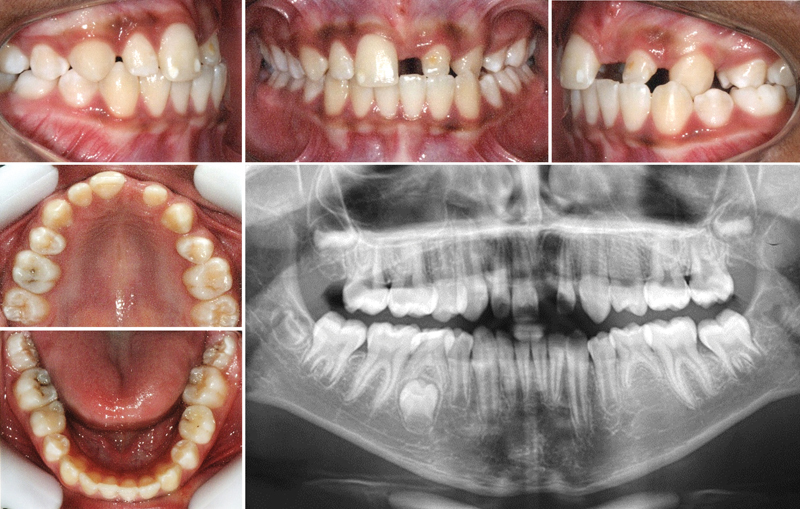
Initial intraoral photographs and panoramic radiograph (of a 11.2-year-old) with avulsion of the maxillary left central incisor and agenesis of the maxillary and mandibular premolars except the mandibular right second premolar.
